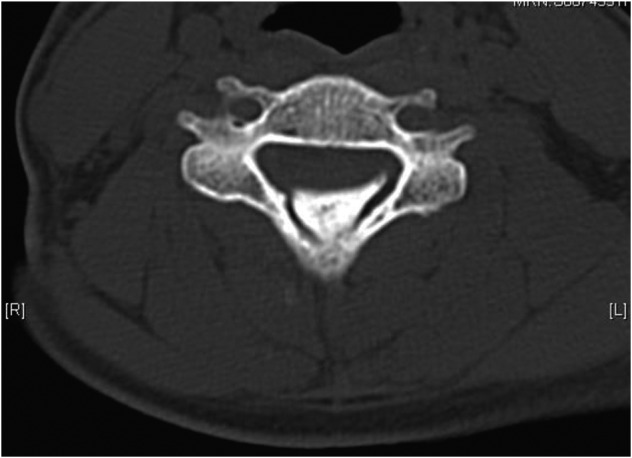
Axial computed tomography image showing thickened nodular ossified yellow ligament centred at C5 level.
